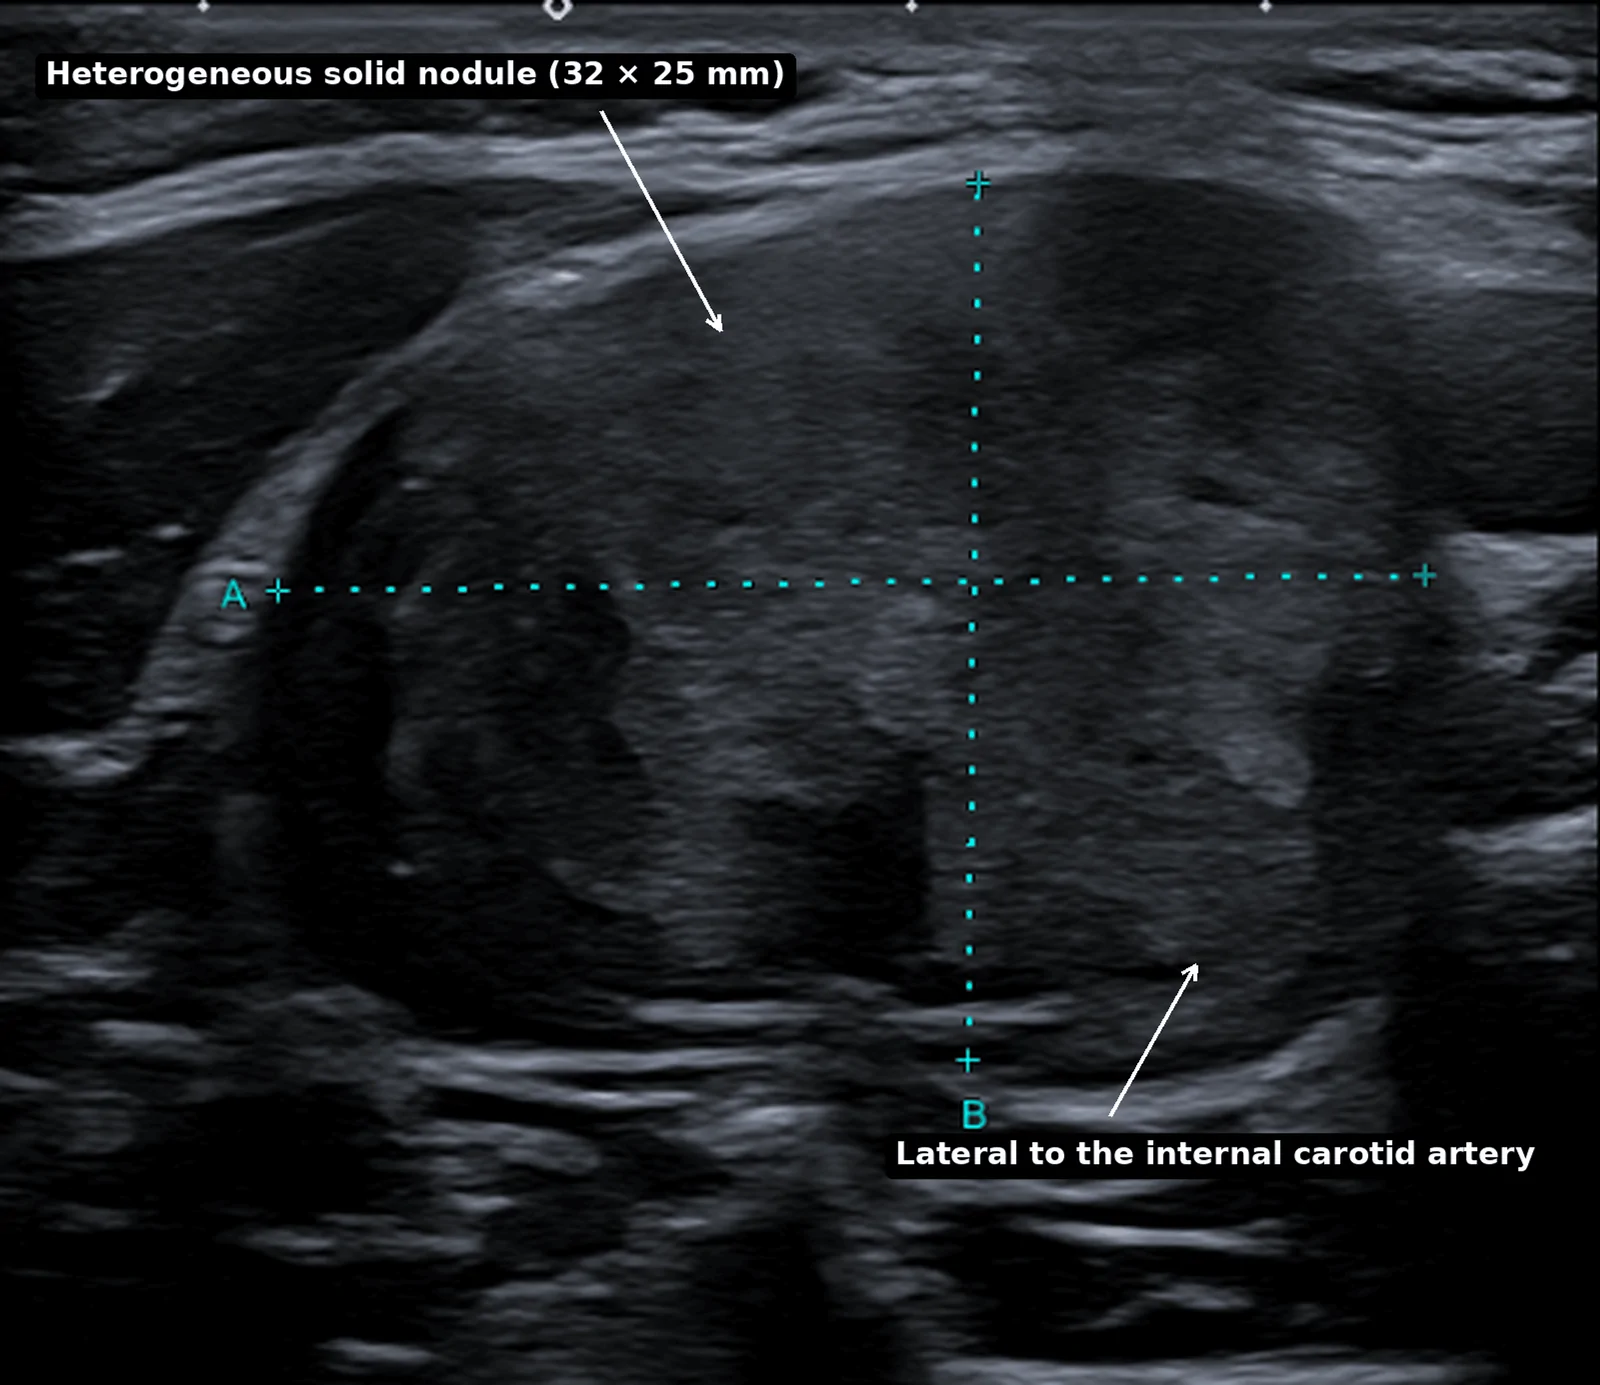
USG of focal heterogeneous solid nodule in the right neck lying just lateral to the internal carotid artery measuring 32 x 25 mm. Ultrasonographic image of the right neck demonstrating a well-defined heterogeneous solid nodule measuring 32 × 25 mm, situated lateral to the internal carotid artery, corresponding to the cervical mass identified on MRI.
